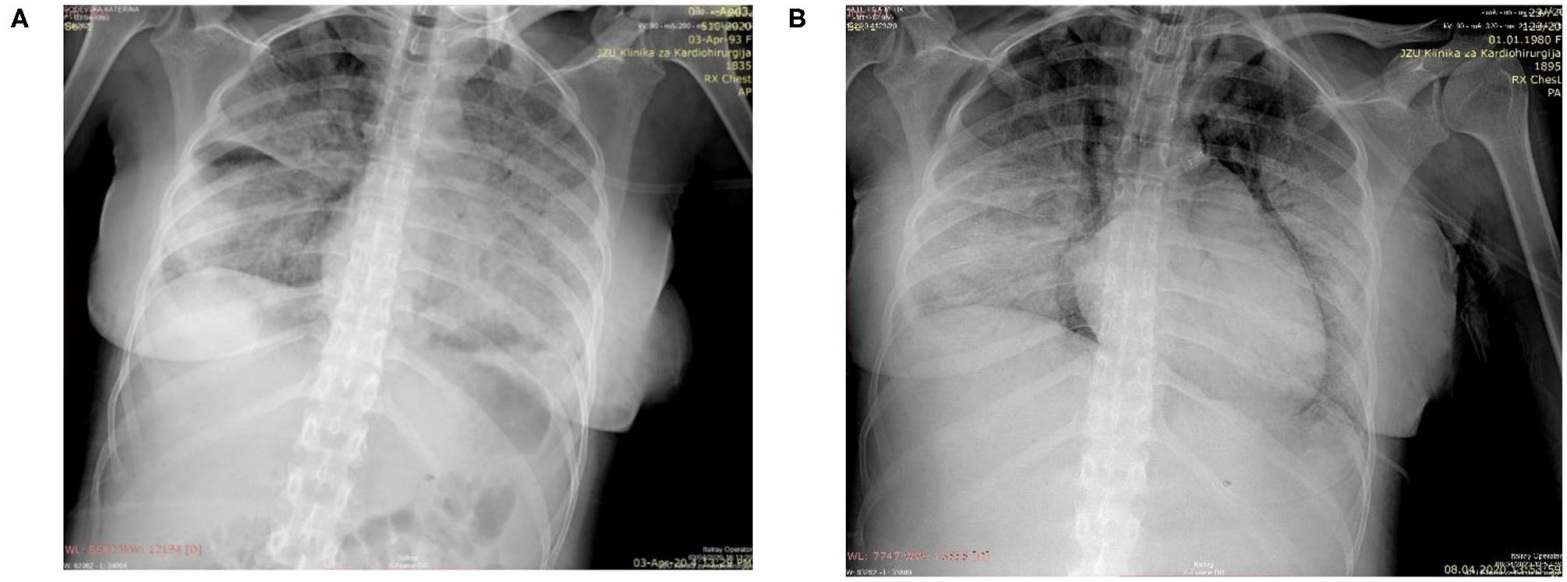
(A) Chest radiograph on day of delivery showing extensive diffuse bilateral opacities suggestive of pneumonia; (B) Chest radiograph taken following endotracheal intubation on the 6th post-delivery day showing progression of pnuemonia, subcutaneous emphysema, pneumomediastinum, and prominent right heart contours suggestive of right heart failure.
